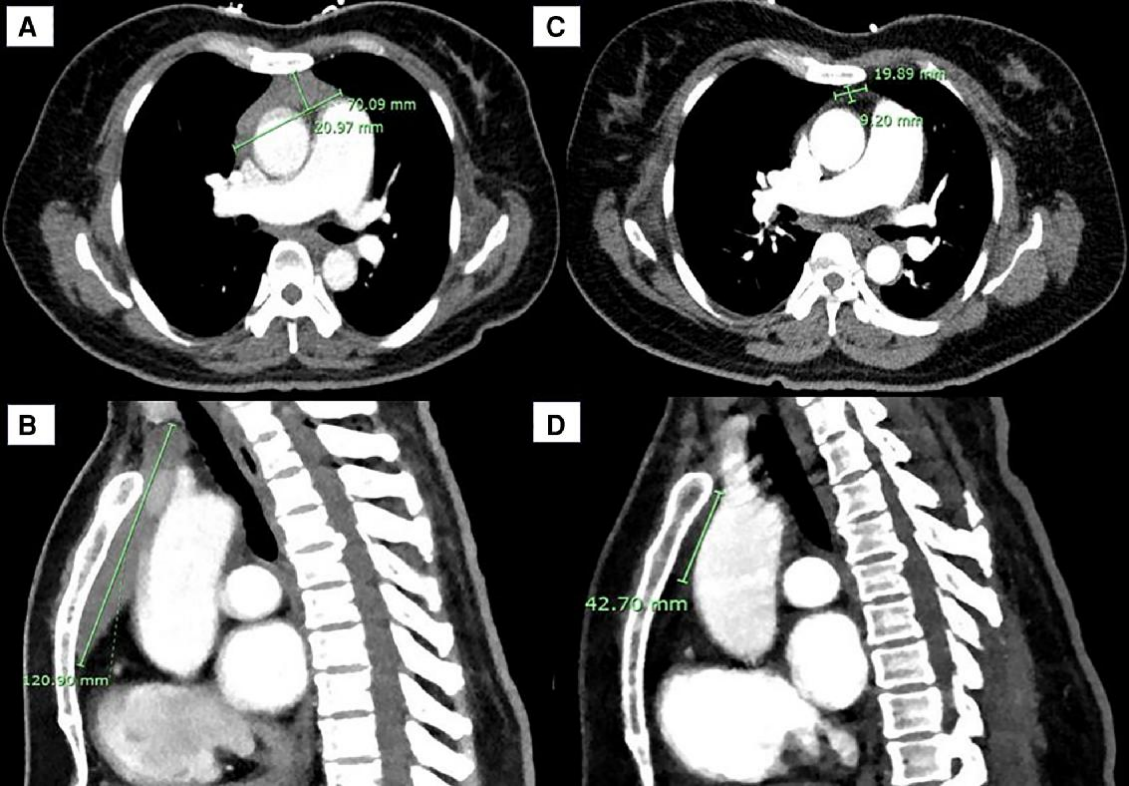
Anterior mediastinal mass (AMM) on computed tomography (CT) of the chest in A, transverse and B, sagittal views on admission to our service, 1 month following onset of Graves disease treatment. Interval CT chest in C, transverse and D, sagittal views 1 year after initial imaging, demonstrating regression of the thymic mass.
